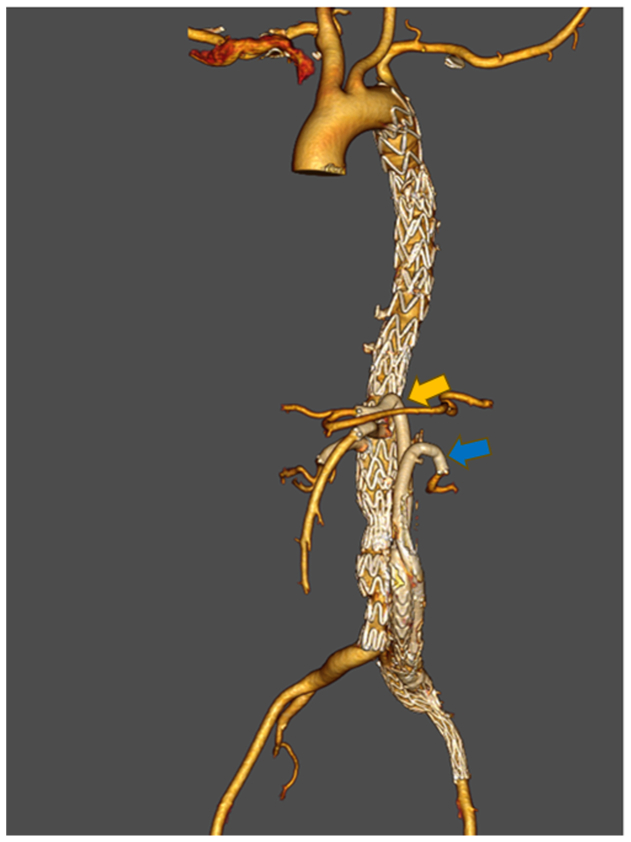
One-year follow-up computed tomography angiography demonstrating complete false lumen thrombosis. *Arrows* indicate patent Viabahn stent grafts in the retrograde-reconstructed celiac trunk (*yellow*) and left renal artery (*blue*).
